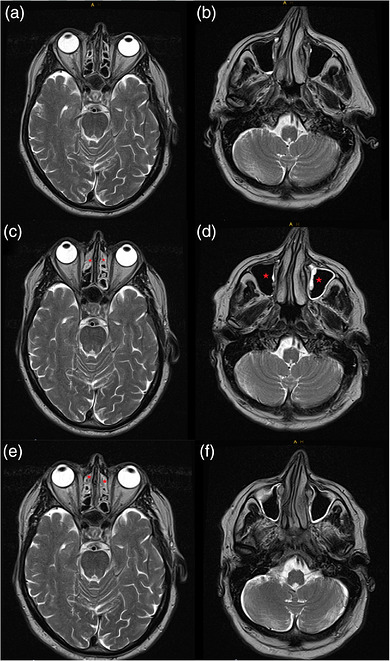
Axial T2‐weighted MRI images of Patient 1 showing (a, b) unremarkable findings prior to initiation of immune checkpoint inhibition; (c, d) symmetrical mucosal thickening of the anterior and posterior ethmoid cells under ICI therapy (red asterisks); (e, f) marked regression of inflammatory changes after systemic and topical corticosteroid therapy.
